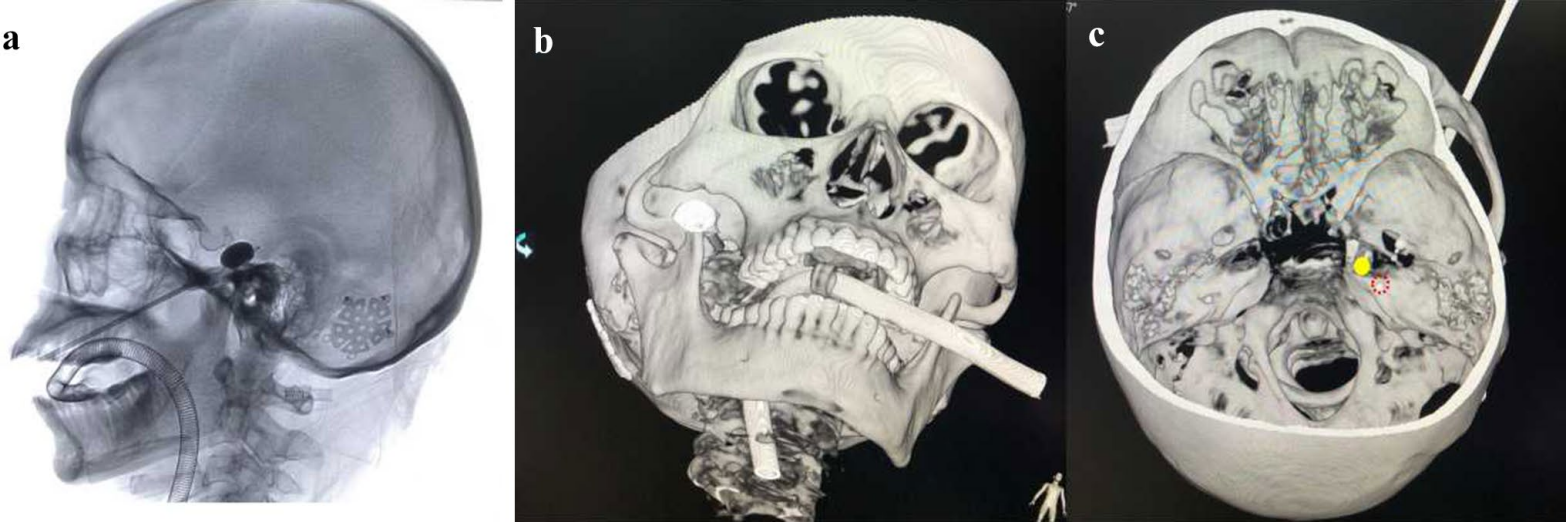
Inaccurate position of balloon catheter before adjustment. a The balloon shape is cylindrical on lateral radiography. b The tip of puncture cannula is projected on the lateral parts of the external opening of foramen ovale from external appearance of skull base in 3D reconstructed CT imaging. c The tip of balloon catheter (red dotted circle) is deviated from the trigeminal porus (yellow solid dot) from internal appearance of skull base in 3D reconstructed CT imaging (color figure online)
